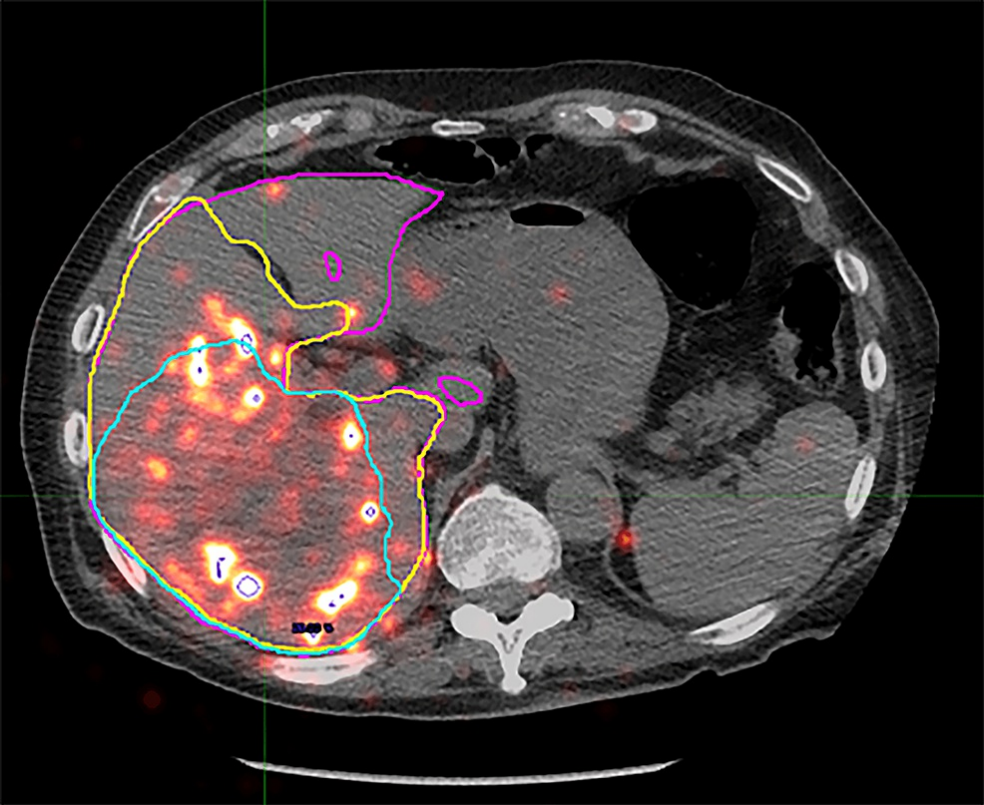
Representative cross-section of operator-defined contours projected on fused 90Y PET/CT Shown are the total liver (magenta), perfused territory (yellow), and tumor (cyan) contours.  The nontumor perfused and unperfused contours are derived from these operator-defined contours by Boolean operation in the software.
